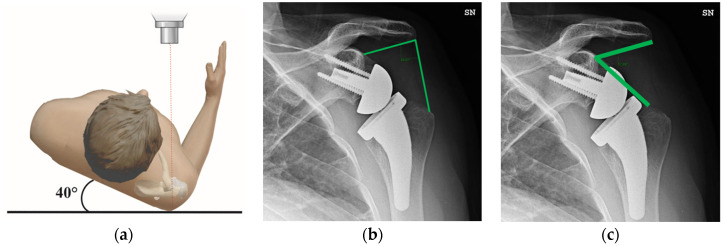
(a) True anteroposterior Grashey view radiography. This requires placing the patient in front of the X-ray beam with the glenoid rim tangential to them, so the shoulder is placed in an ipsilateral posterior oblique position. Radiographic indexes measured on anteroposterior Grashey view; (b) lateralization shoulder angle (LSA); (c) distalization shoulder angle (DSA).
